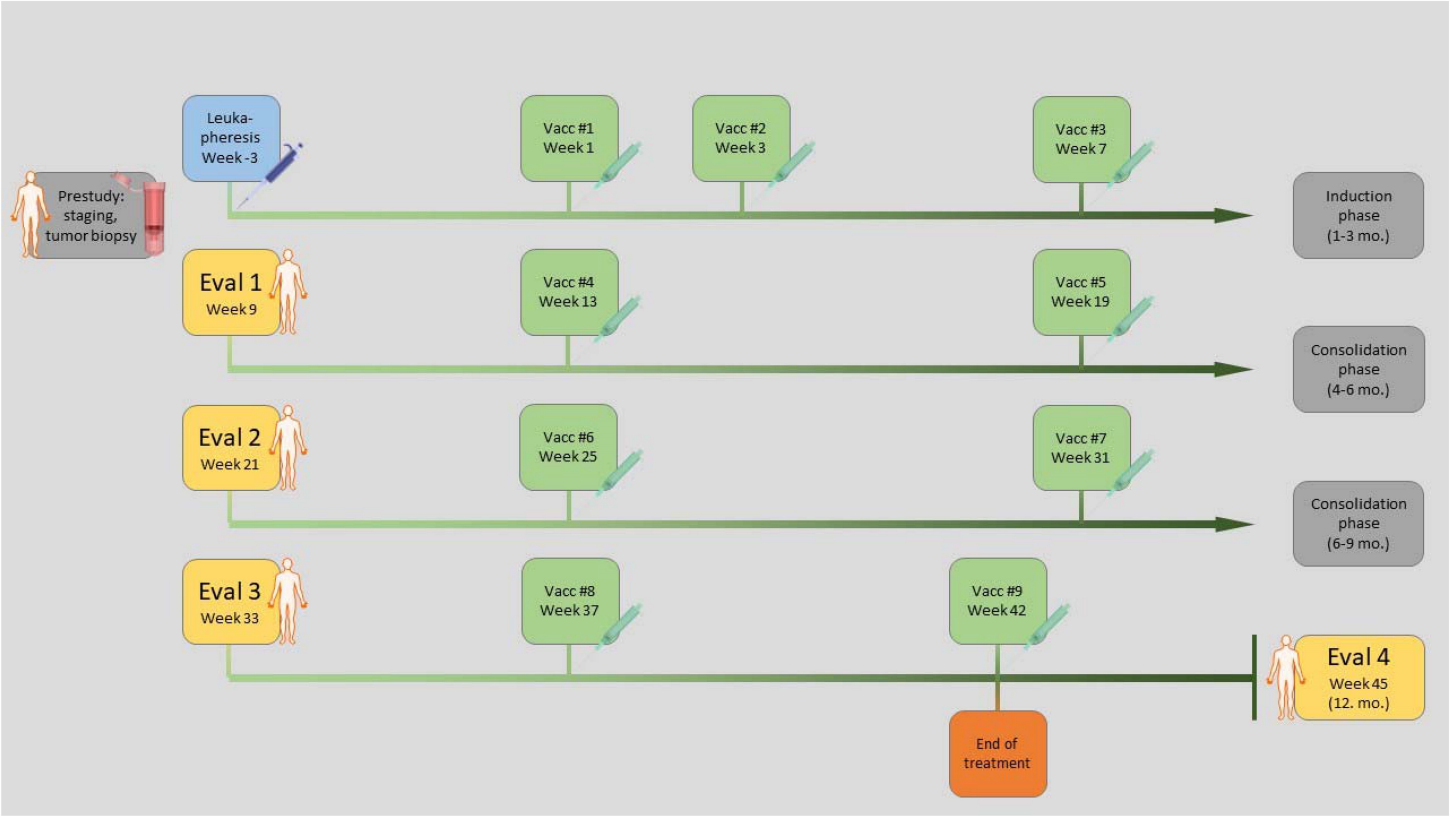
Treatment schedule: Initially a tumor biopsy is taken, and RNA and DNA preparation and sequencing is performed. To obtain the required numbers of autologous DCs, a monocyte concentrate is collected *via* leukapheresis. The vaccine is generated by RNA-electroporation of DCs and 9 vaccine injections are given intravenously over a period of one year. Every three months CT/MRI staging is performed.
